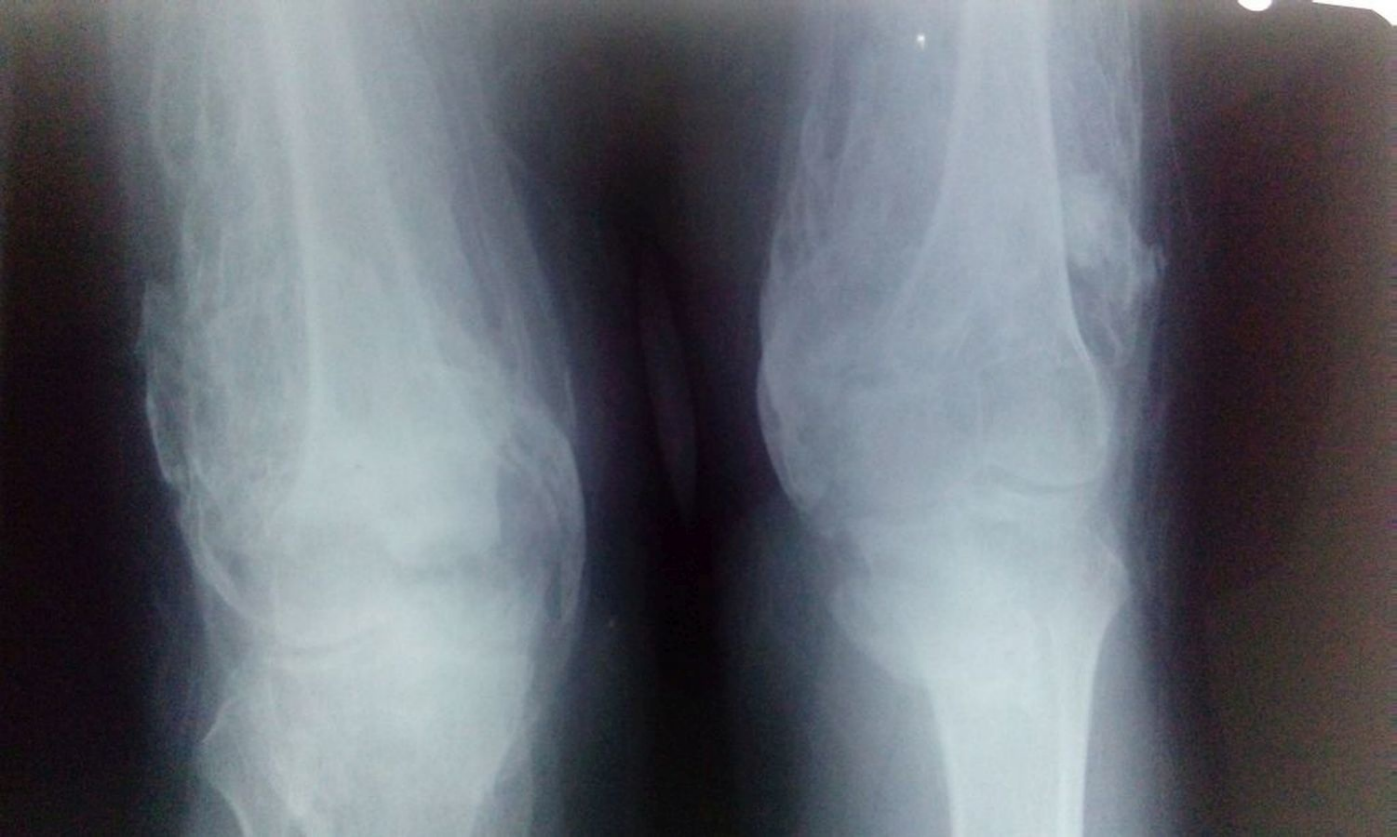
Anteroposterior radiograph of the knees showing diffuse heterotopic ossification
